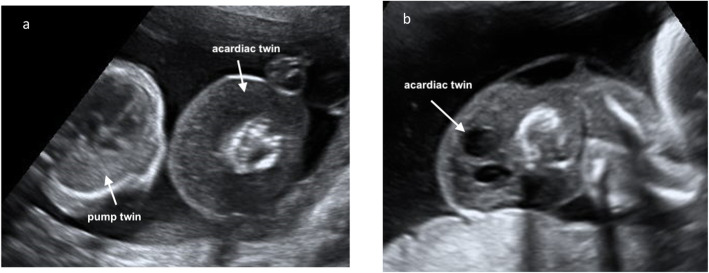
Ultrasound images of a twin reversed arterial perfusion (TRAP) sequence. a Transverse view of both the pump and acardiac twin, *white arrows*. b*White arrow*, longitudinal view of the acardiac twin
